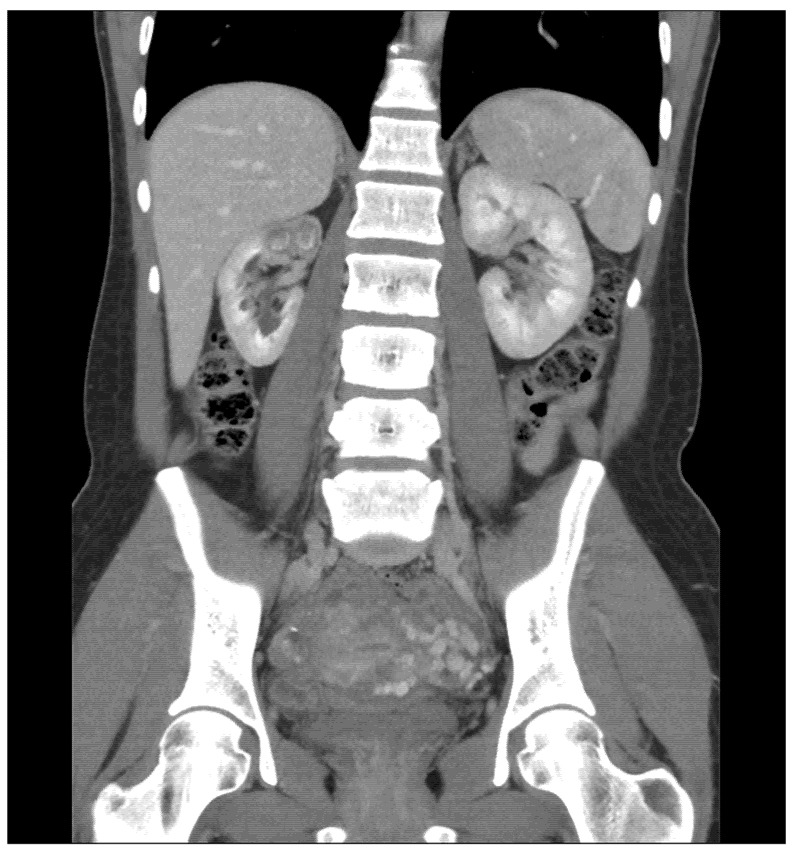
CT Scan image showing right upper pole kidney TB lesions—coronal view.
